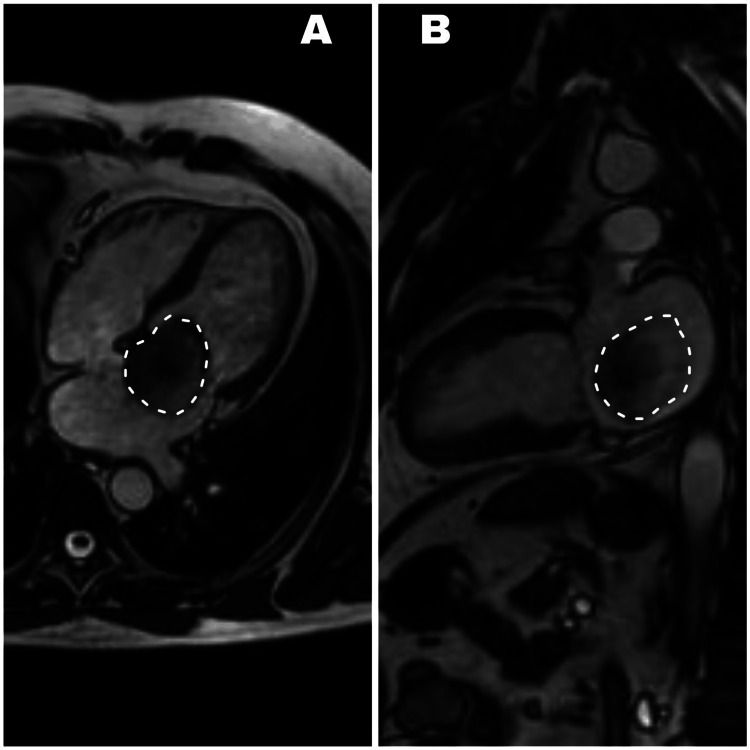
Cardiac Magnetic Resonance Imaging A mobile, oval-shaped mass measuring 55 x 39 mm, adherent to the interatrial septum, is identified. A. SSFP sequence, four-chamber view during diastole, demonstrates a left intra-atrial space-occupying lesion with apical displacement toward the left ventricular inflow tract, resulting in partial obstruction. B. SSFP sequence, two-chamber view during diastole, reveals a left intra-atrial space-occupying lesion. SSFP, steady-state free precession.
